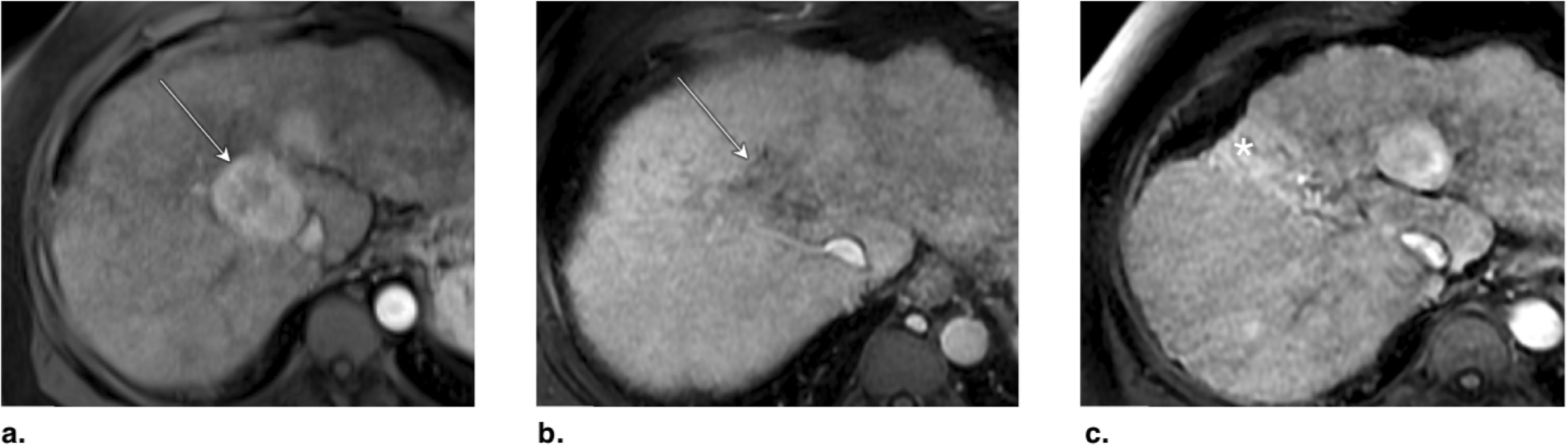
Axial T1-weighted MR obtained (a) pretreatment: image in late arterial phase (AP) shows a 5-cm LR-5 observation (arrow). b 1 month post transarterial radioembolization (TARE): image in portal venous phase shows hypoenhancing area. c 1 year post TARE: image in late AP shows expected capsular retraction and perfusional anomalies (asterisk). The treated observation is categorized LR-TR Nonviable
